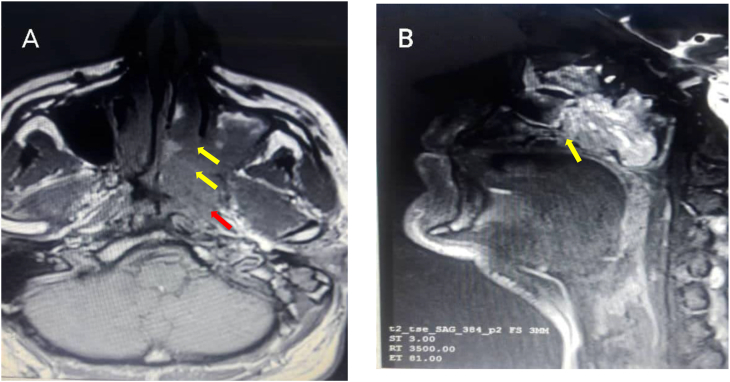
MR images showing a heterogeneously enhancing tumor in the maxillary sinus. A: the tumor eroded the medial wall of the sinus (yellow arrows) and reached the pterygopalatine fossa (red arrow). B: the tumor eroded the posterior aspect of the hard palate (yellow arrow). (For interpretation of the references to colour in this figure legend, the reader is referred to the Web version of this article.)
